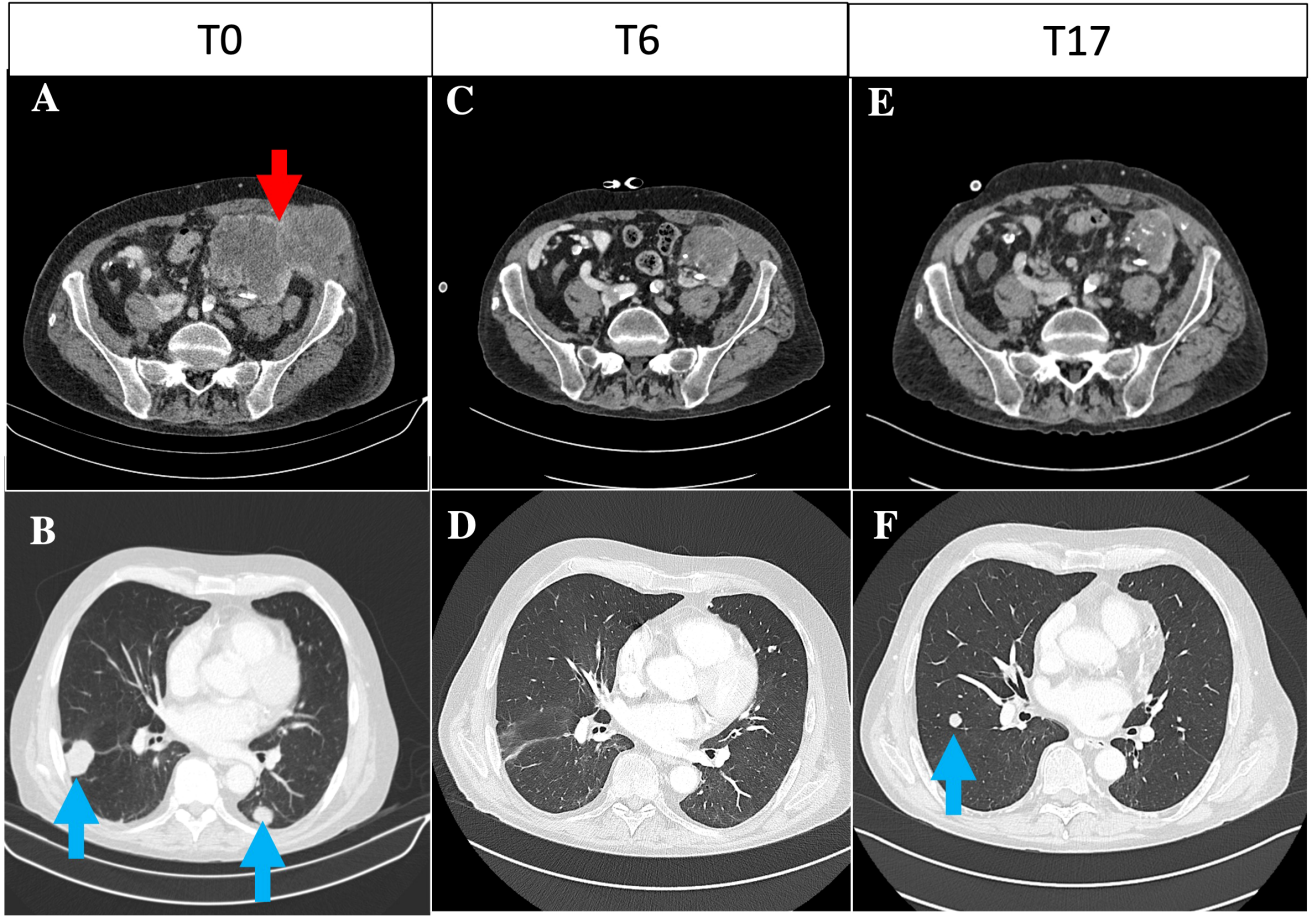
(A, B) Basal contrast-enhanced CT of the pelvis and thorax at baseline. (A) Lobulated contrast-enhanced expansive lesion of approximately 12 cm in the left graft with extension in the context of the abdominal wall muscles (71 × 63 mm, *red arrow*). (B) Multiple parenchymal nodulations in both lungs suggestive for metastases, the largest of approximately 18 mm in the right lower lobe (*blue arrows*). (C, D) Contrast-enhanced CT of the pelvis and thorax at baseline 6 months post-immune checkpoint inhibitor (ICI) initiation. (C) Lobulated expansive lesion in the left graft (50 × 46 mm), with extension in the context of the abdominal wall muscles (42 × 22 mm). (D) Overall reduction of multiple parenchymal nodulations in both lungs. (E, F) Contrast-enhanced CT of the pelvis and thorax at baseline 17 months post-ICI initiation. (E) Stable lobulated expansive lesion in the left graft (43 × 39 mm), with extension in the context of the abdominal wall muscles (40 × 18 mm). (F) Overall lung progression, with increase of some pulmonary nodules, the largest of 14 mm at the upper left lobe and 15 mm at the right lower lobe.
